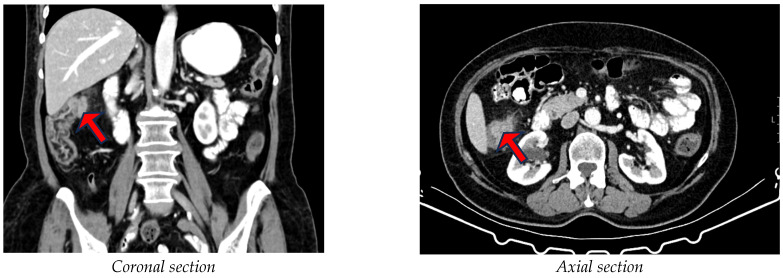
Abdominal CT scan showing colonic wall infiltration. Arrows show T3 tumor in coronal and t3 tumor in axial section.
